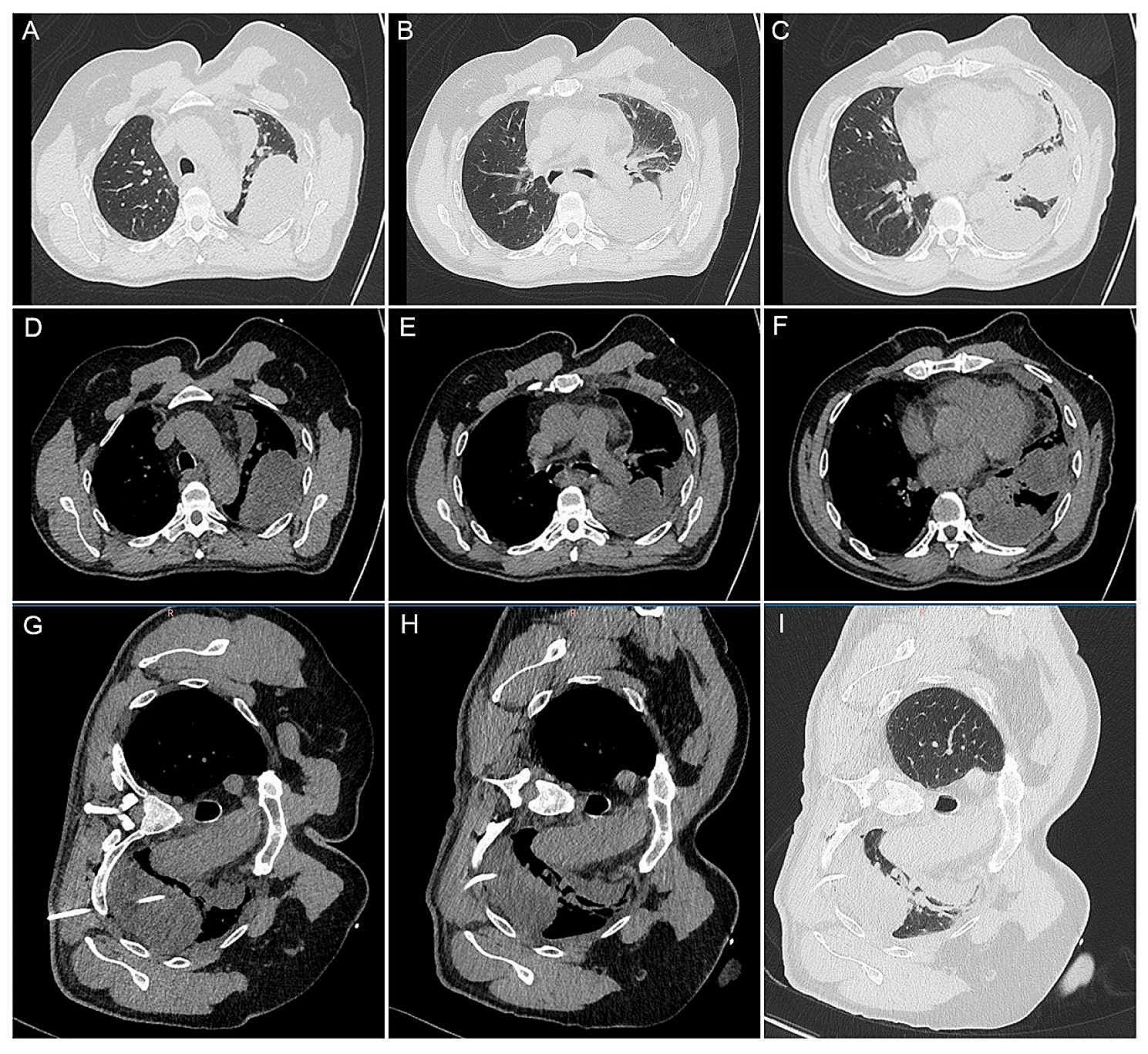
Chest radiological signs of the patient. (A-F) Chest CT showed encapsulated pleural effusion on admission. (G-I) 8 F pigtail catheter (NNU8LPT, Bard Access Systems, Inc) was placed into left side pleural effusion guided by CT scan
